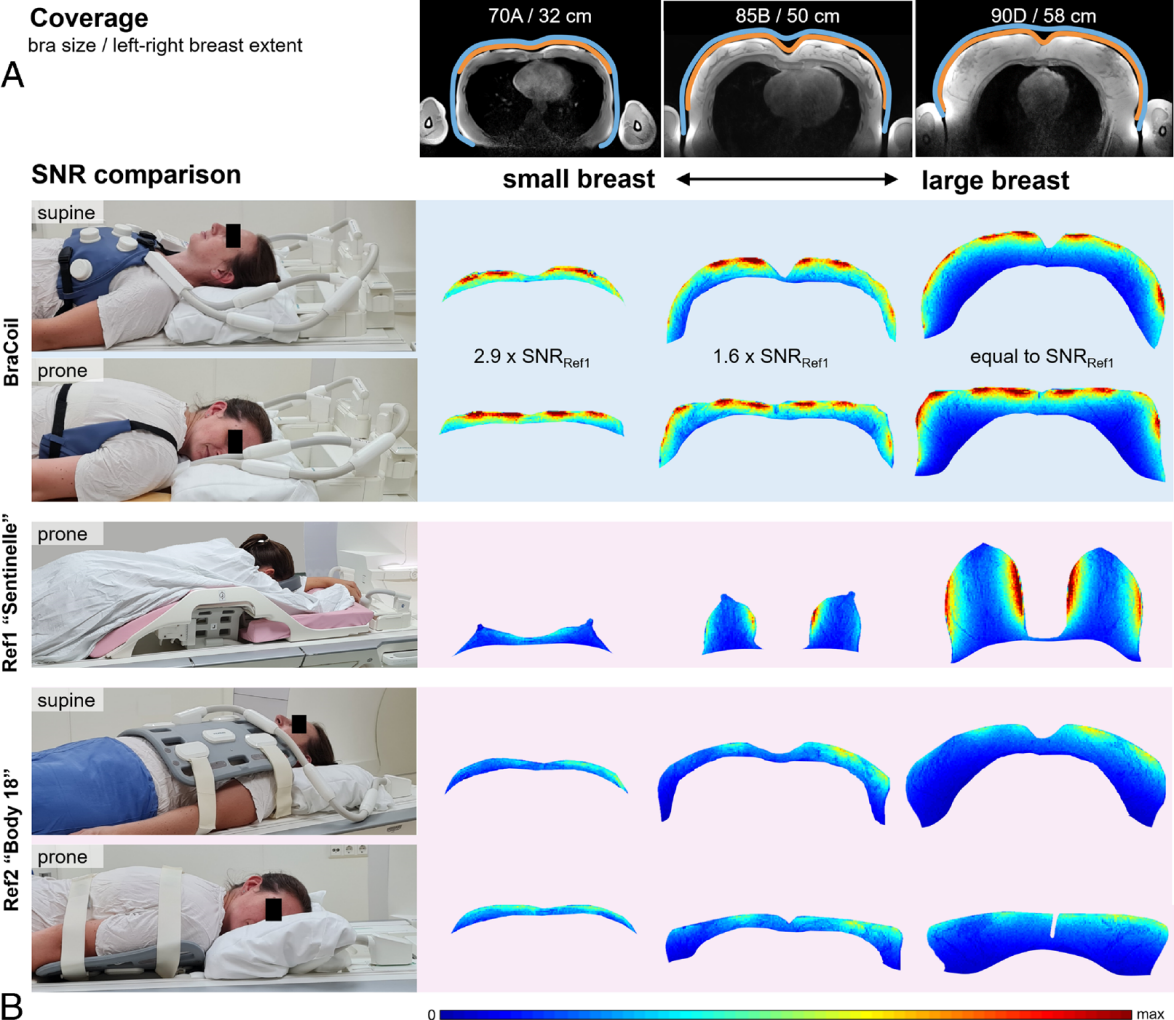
Performance of the BraCoil for different breast sizes and comparison to the clinical standard reference coils. The spatial distribution of SNR achieved with the BraCoil and the 2 reference coils is displayed, demonstrating that the BraCoil achieves higher SNR, particularly for smaller breasts. The flexibility of the coil ensures a tight fit and sufficient coverage for small and large breasts alike. A, Axial T1-weighted gradient echo images show the sensitive area (coverage) of the coil. The blue curves indicate the approximate position of the coil, orange curves indicate the left-right extent of the breasts measured along the body surface. Bra sizes and left-right extent of the breasts are indicated. B, SNR maps are shown for the BraCoil in supine and prone positioning of the patient, in prone position for reference coil Ref1 “16-channel Sentinelle Breast Coil,” and in supine and prone position for reference coil Ref2 “Body 18.” SNR was evaluated in the whole breast volume and nonbreast tissue was removed for display purposes.
